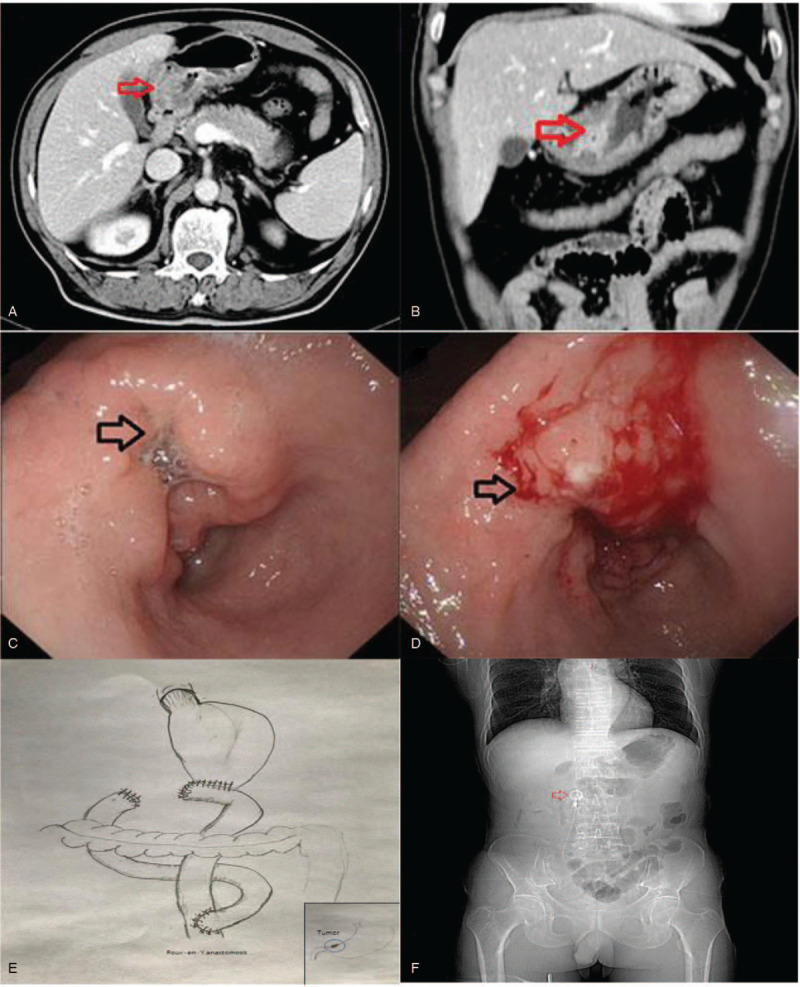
Preoperative abdomenal enhanced computed tomography (CT) test and endocopy. A: cross-section CT examination showed the gastric antrum tumor invaded the surrounding structures; B: coronary CT examination revealed a large mass in the gastric antrum in the stomach and obstruction; C: gastroscopy showing a large irregular ulcer in the antrum; D: ulcerative bleeding after gastroscopy. E: Roux-en-Y anatomosis after gastrecromy. F: X-ray of abdmen shows peritoneal pump.
